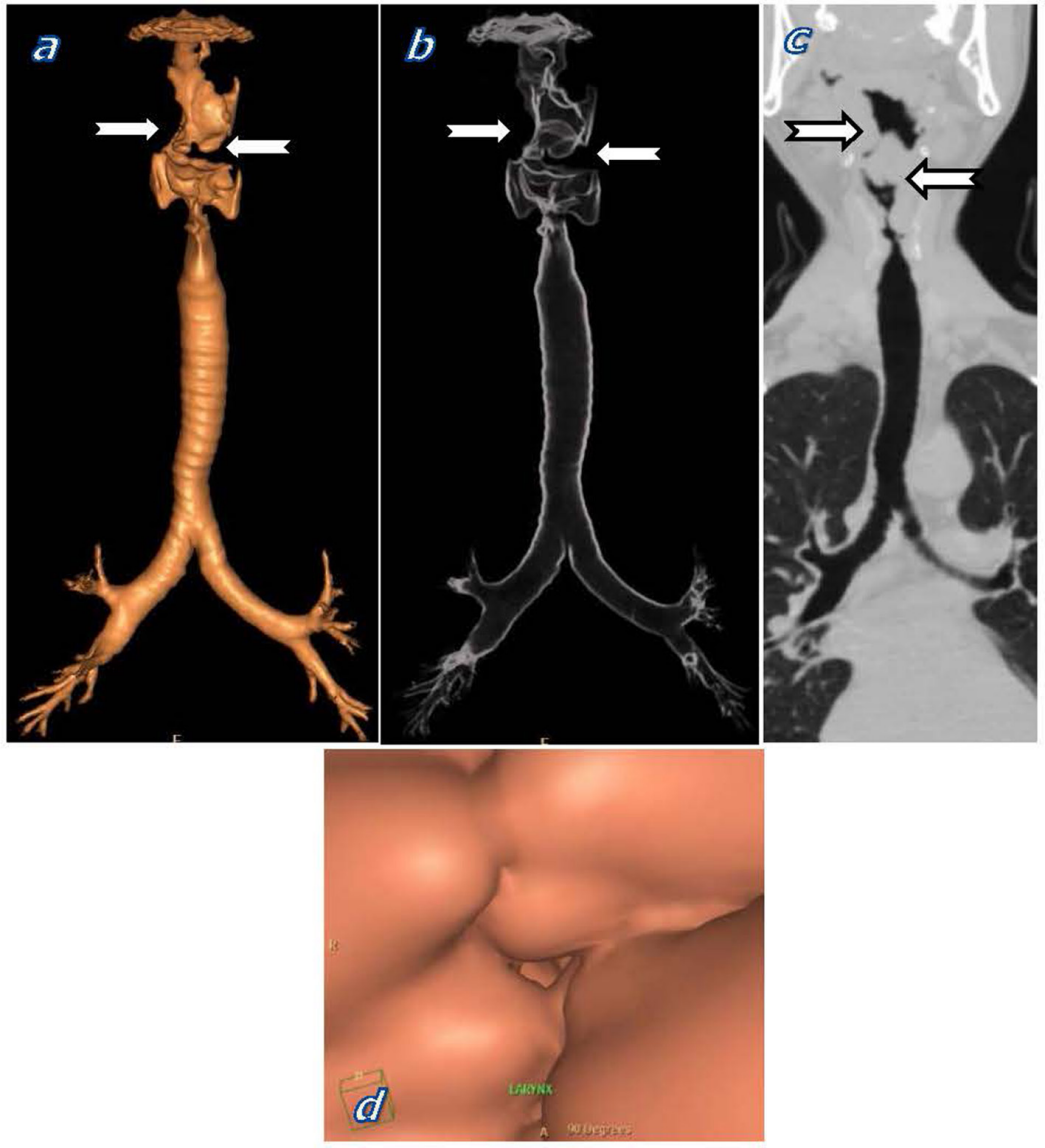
Volume-rendered 3D CT virtual bronchoscopy, (b) 3D transparency view, (c) coronal MPR, and (d) endoluminal view showed laryngeal lumen narrowing and irregular nodular thickening of the laryngeal wall (notched arrows). Normal appearance of the tracheobronchial tree. 3D, three-dimensional; MPR, multiplanar reconstruction.
